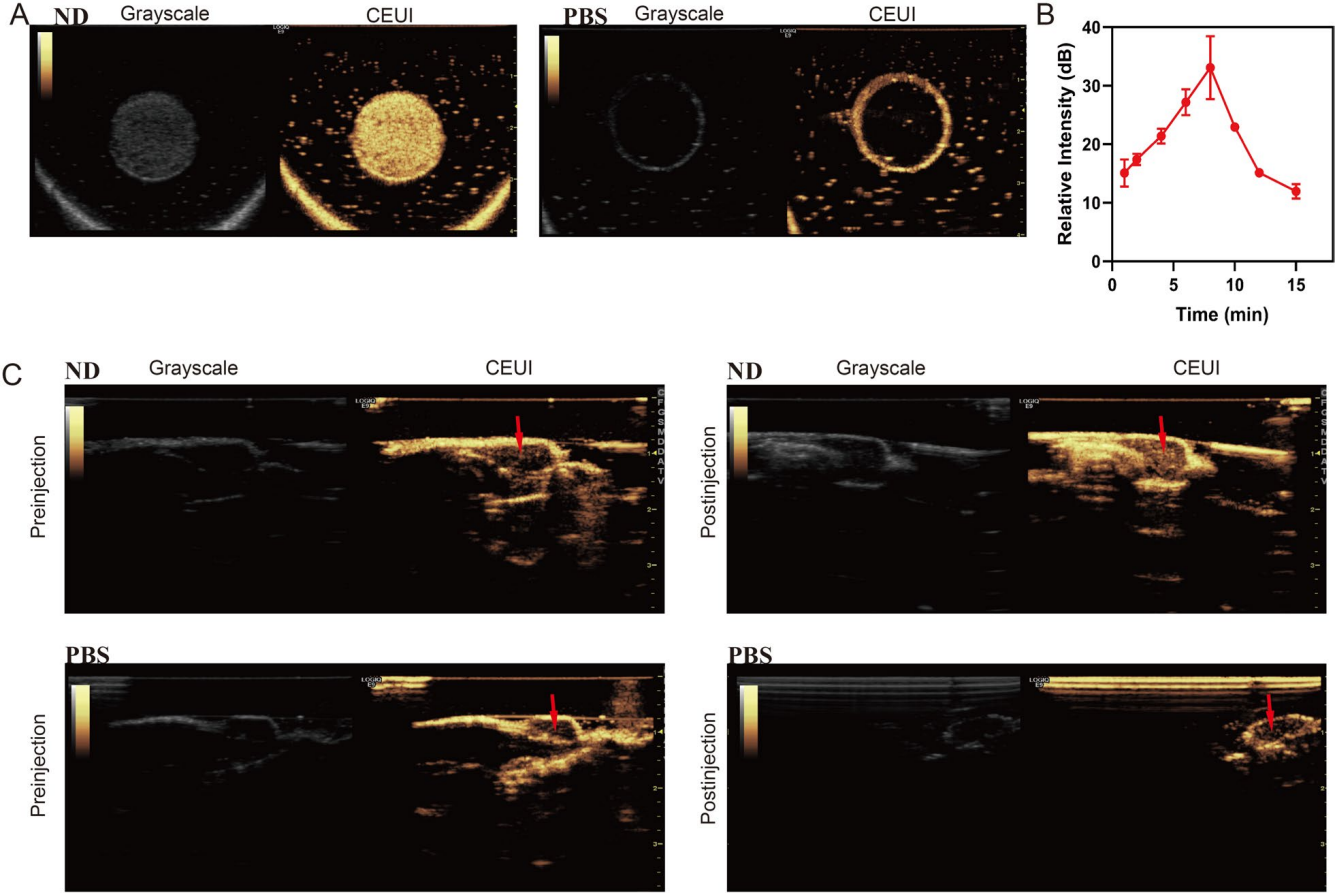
CEUI of NDs. A Ultrasound imaging in vitro. B TIC in tumors after administration of NDs in vivo. C Ultrasound imaging in vivo. The red arrows pointed to the tumor sites
